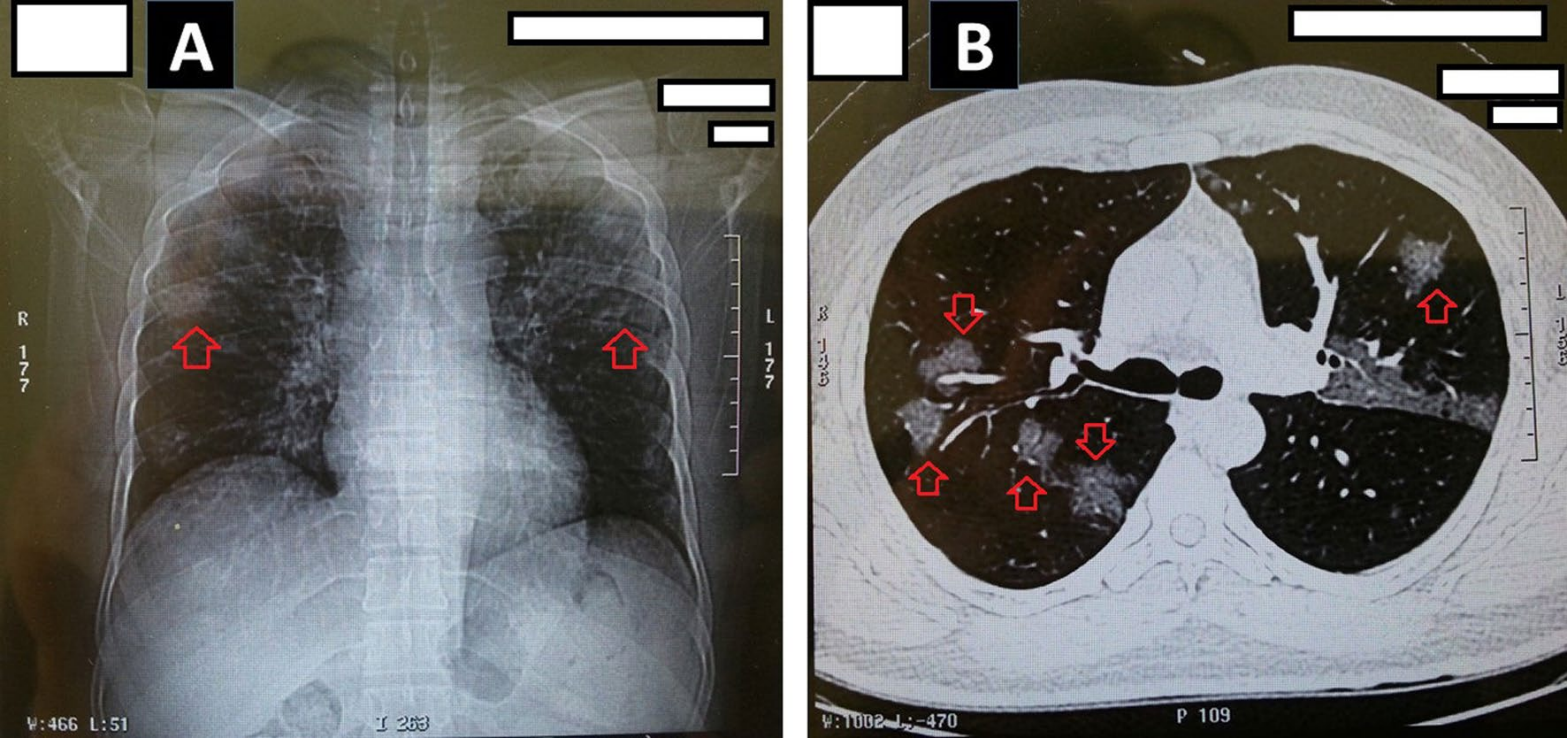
Chest computed tomography (CT) scan in the reported case with COVID-19. A Ground-glass opacification (GGO) in COVID-19 pneumonia; B bilateral and multilobar GGO in lungs. Arrows show the GGO in lungs
